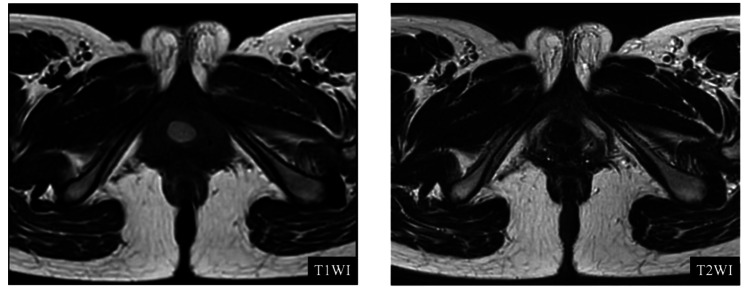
MRI findings (T1- and T2-weighted images) Axial images showed a well-defined cystic lesion lateral to the distal urethra. The lesion demonstrated high signal intensity on T1-weighted imaging and low signal intensity on T2-weighted imaging, with a thin low-signal rim delineating the cyst wall. No communication with the urethra or solid components was observed.
